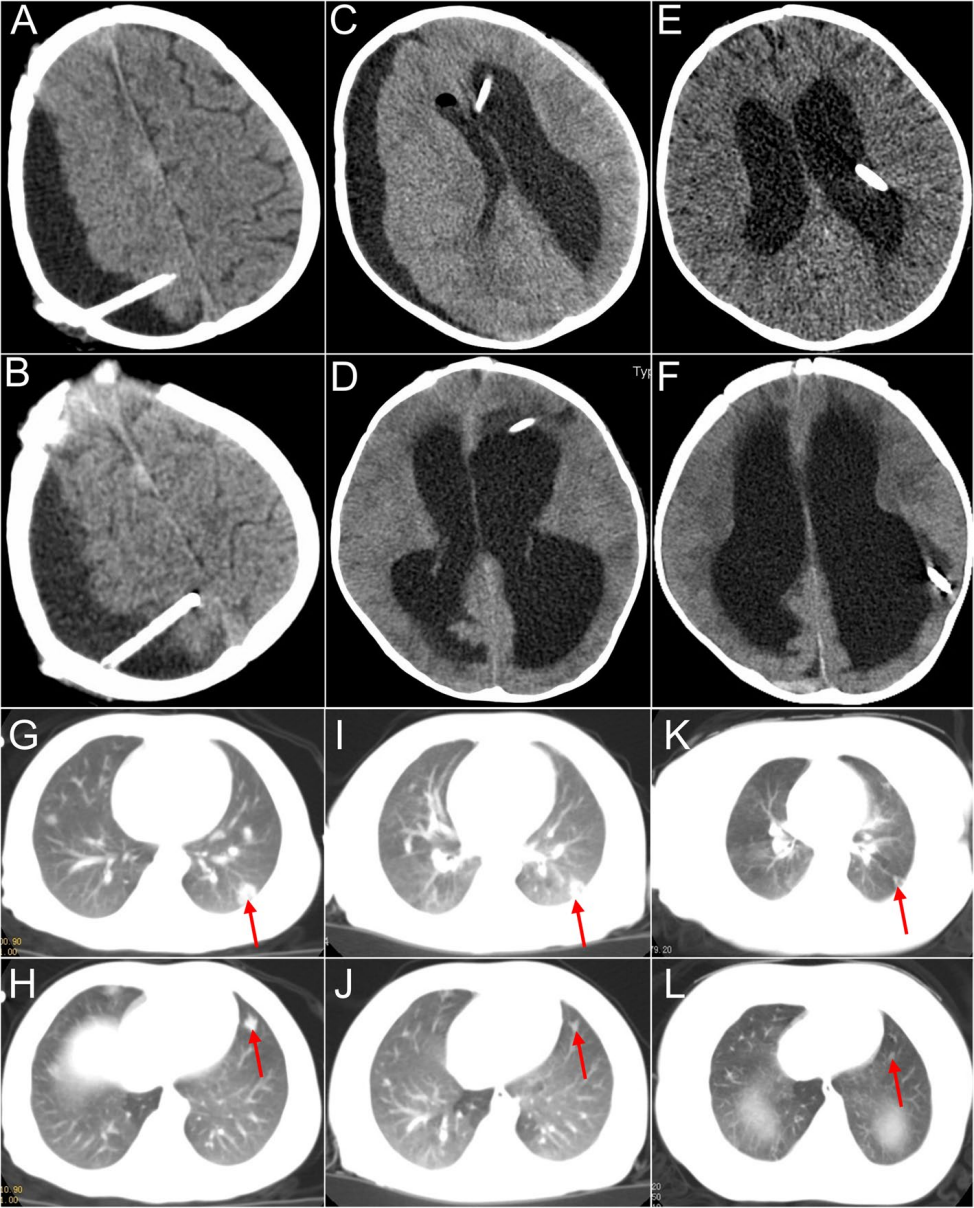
The management of CSF and the lung CT during chemotherapy. The SDE did not relieve on day 1 (A) and day 7 (B) after burr hole drainage. SE gradually relieved on day 1 (C) and day 7 (D) after implanting the Ommaya reservoir. SE gradually relieved on day 1 (E) and day 7 (F) after implanting a long-distance open ventricular drainage operation. The nodes (red arrow) on the lungs did not shrink one month (G and H) and two months (I and J) after the management of treatment with crizotinib and vincristine. After two months of cytarabine, crizotinib, and vincristine treatment, lesion remission was observed (K and L). The measured resolution for images was 360 ppi
